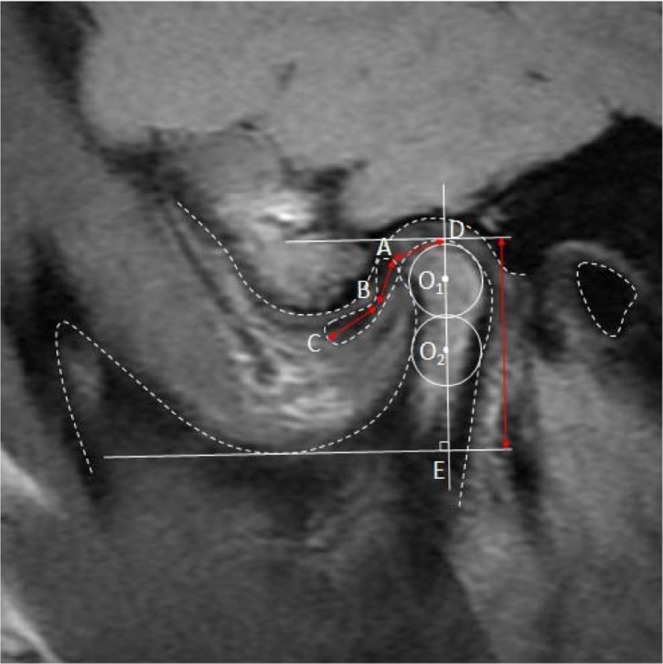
Key reference points on MRI measurement. Point A is located in the posterior border of the disc; point B is located in the centre of the disc; point C is located in the anterior border of disc; point D is the 12-o’clock position on the head of condyle; point E is the intersection of the condyle axis and its perpendicular cutting sigmoid notch. Disc length is the total of the length of line AB and line BC. Line DE shows the height of the condyle.
